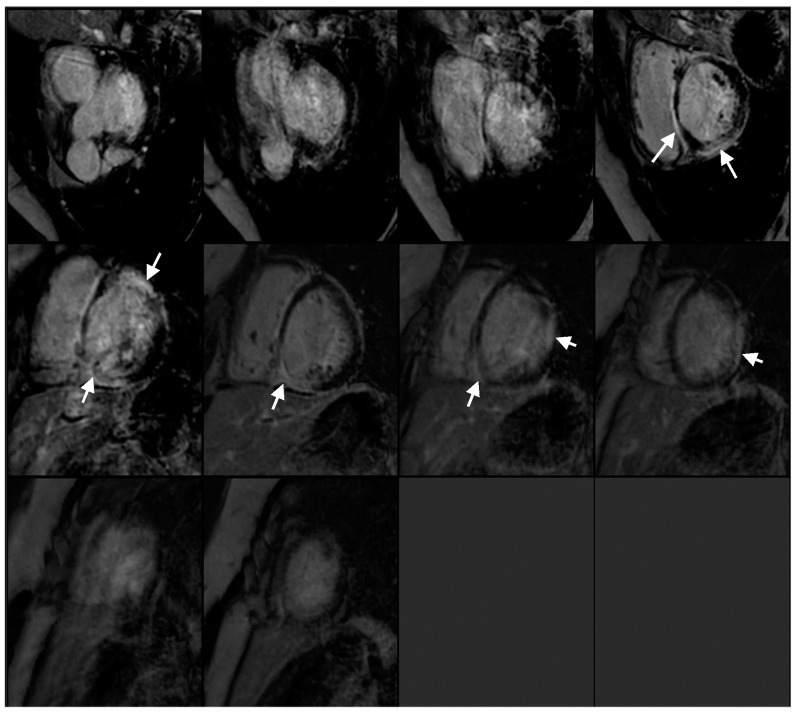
Cardiac MRI with multifocal LGE (arrows) consistent with an inflammatory cardiomyopathy.
